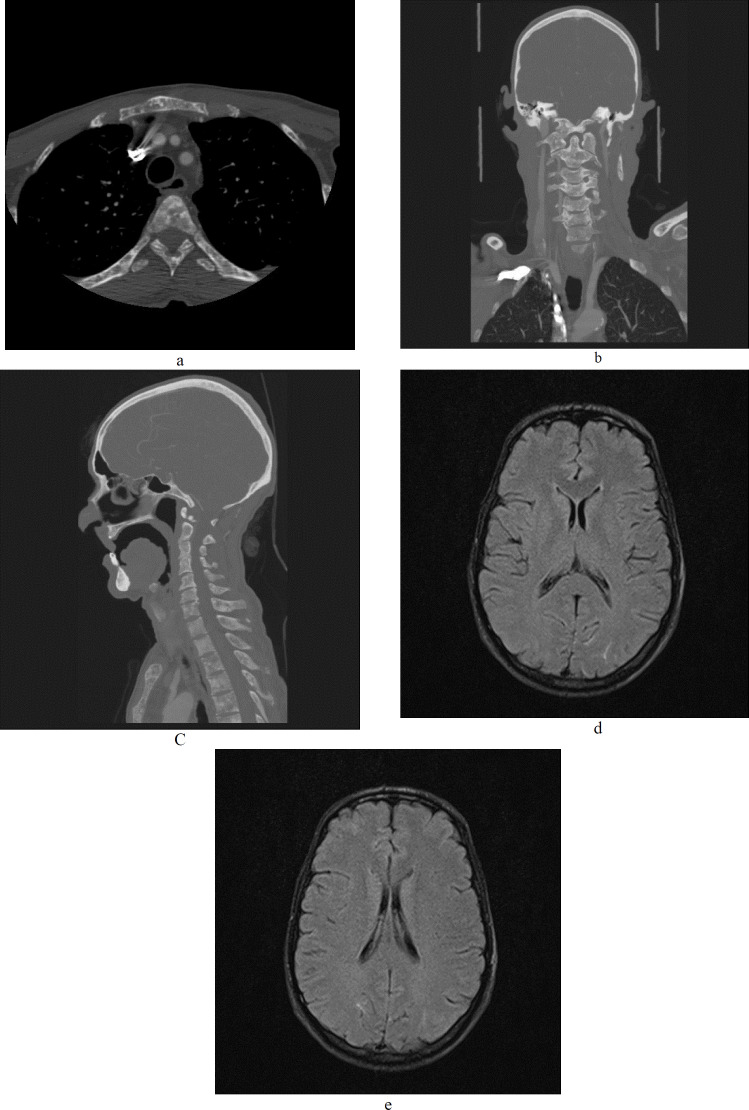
Axial, coronal, and sagittal CT scans of chest and neck showing multiple sclerotic lesions in the vertebral bodies, posterior elements, ribs, and sternum which cause a diffuse inhomogeneous appearance. d, e: Axial FLAIR image of brain showing increased signal intensity in the subarachnoid space and sulci of both occipital lobes
